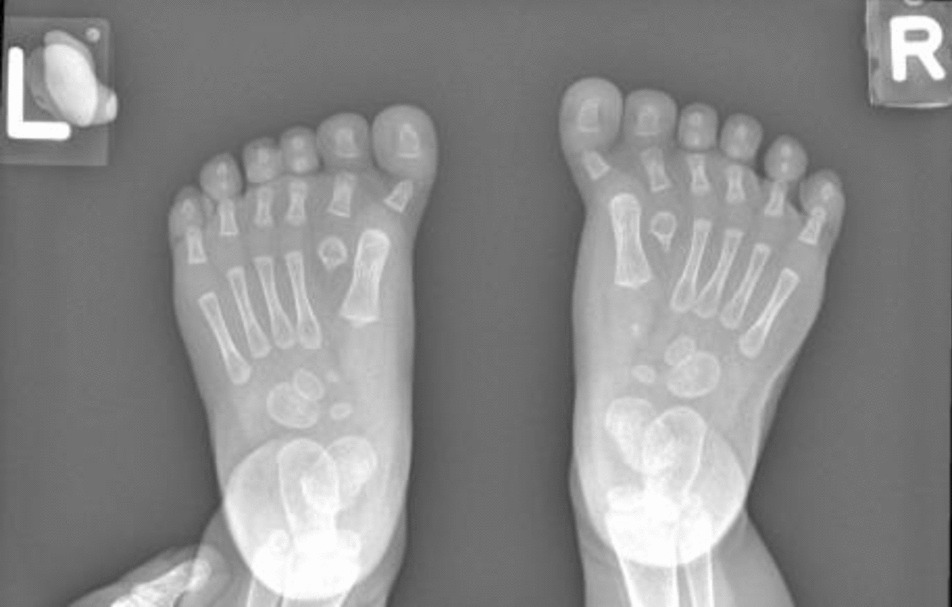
X-ray showing bilateral big toe polydactyly
